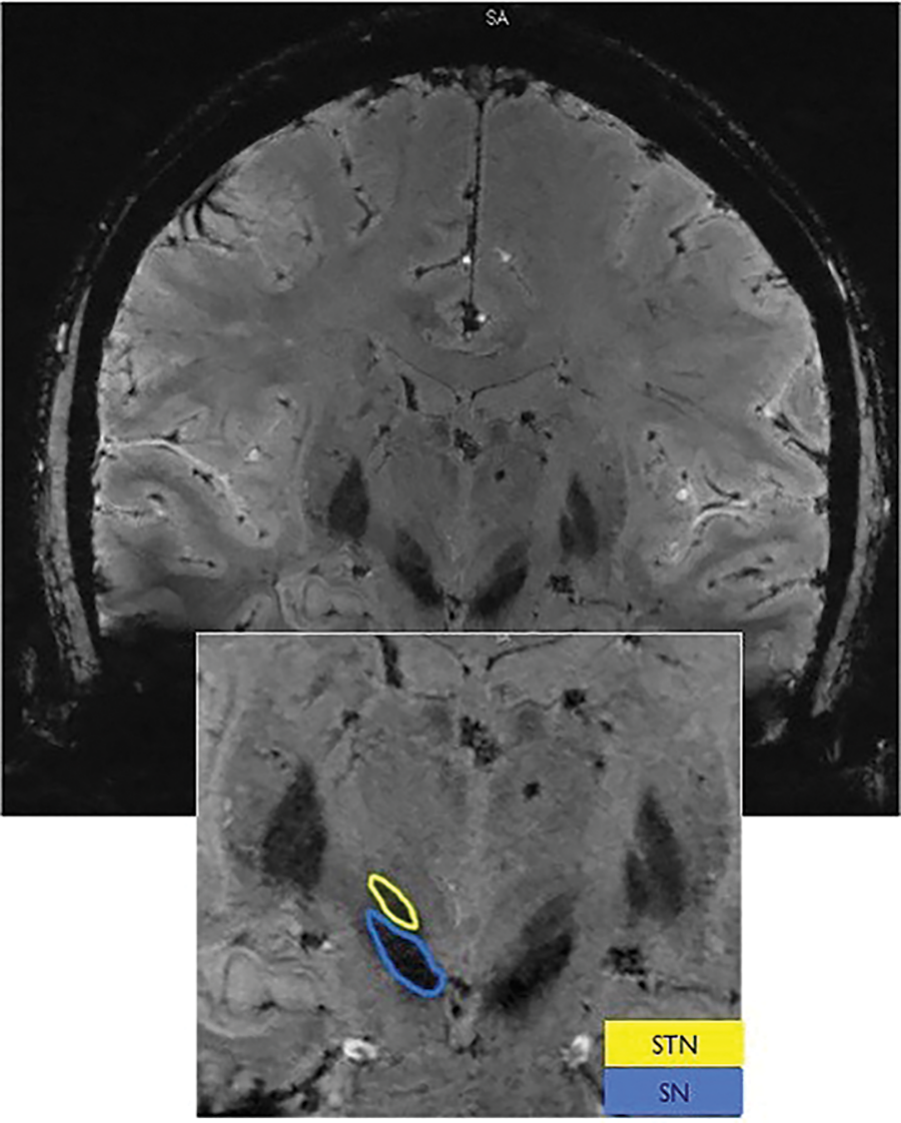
Sub-cortical anatomical structures on High-resolution 7T MR images. High-resolution 7T images are used for visualizing sub-cortical anatomical structures. As an example, the subthalamic nuclei (STN) and substantia nigra (SN) are identified on a coronal view using susceptibility-weighted imaging (SWI) contrast. Note the clear image-contrast separation between the SN and STN that allow segmentation of those structures with high confidence.
